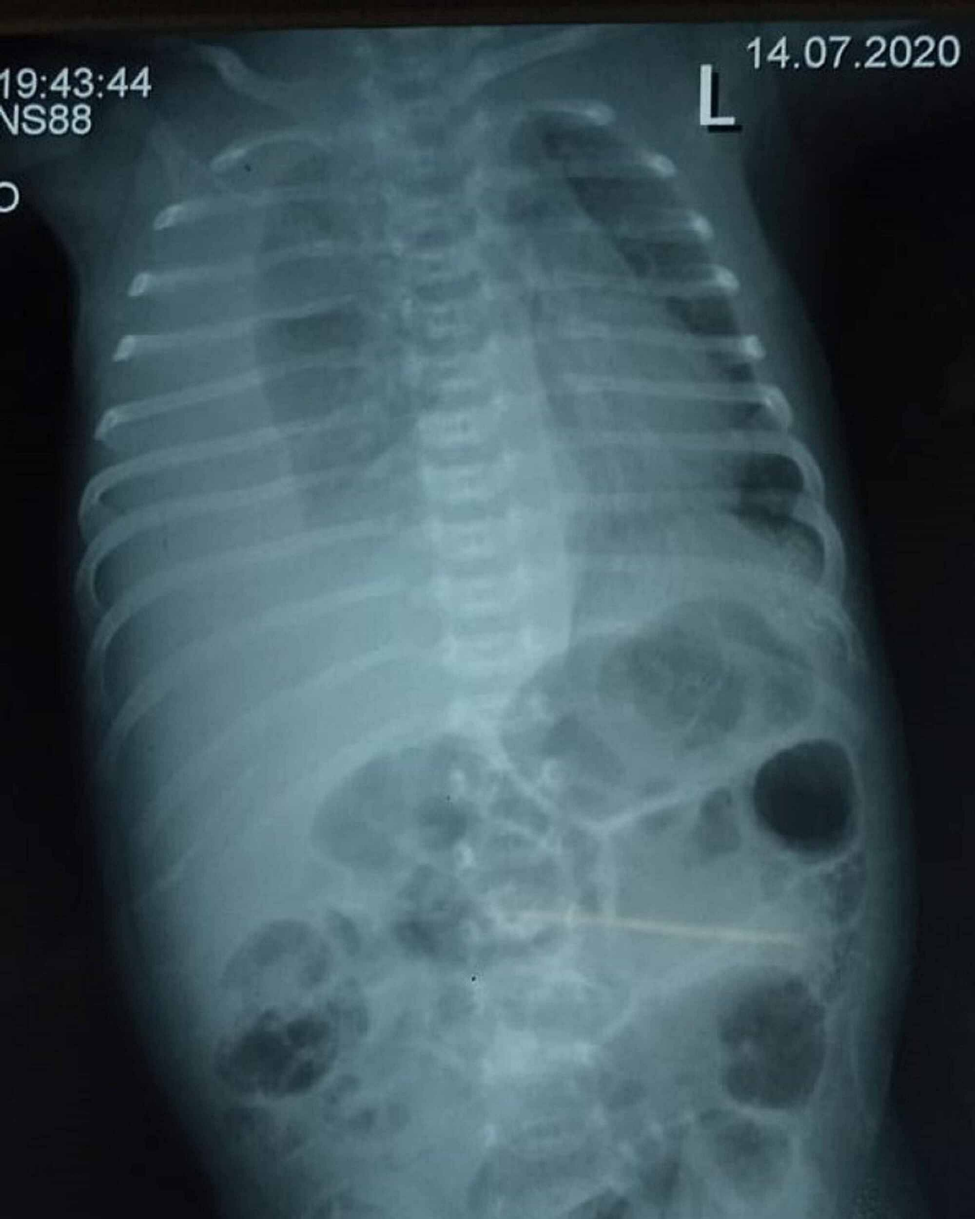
Chest X-ray before pleural tap showing massive right-sided pleural effusion
